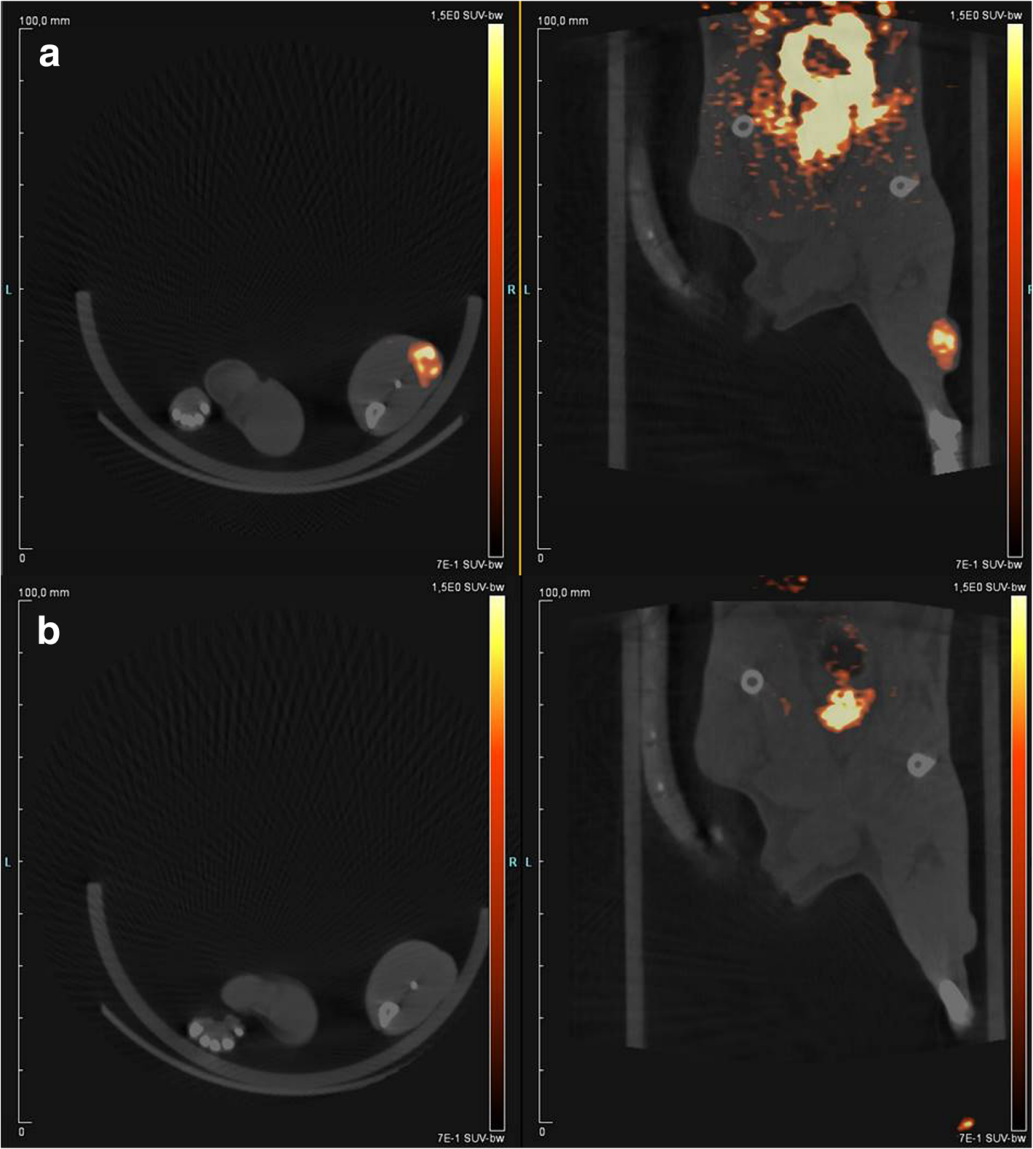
[^18^F]FAZA-PET/CT of a HI-tumor 2 h post tracer injection, before (a) and after single fraction of ^12^C-ions (b) in axial (left panel) and coronar (right panel) image orientations. The PET tracer is color-coded and overlayed to an aligned CT image. Before RT (a), a high tracer uptake was found in the tumor as well as in the bladder due to partly urinary excretion of the tracer. 7 days after RT (b), the same tumor showed a tracer uptake in the bladder but not in the tumor
